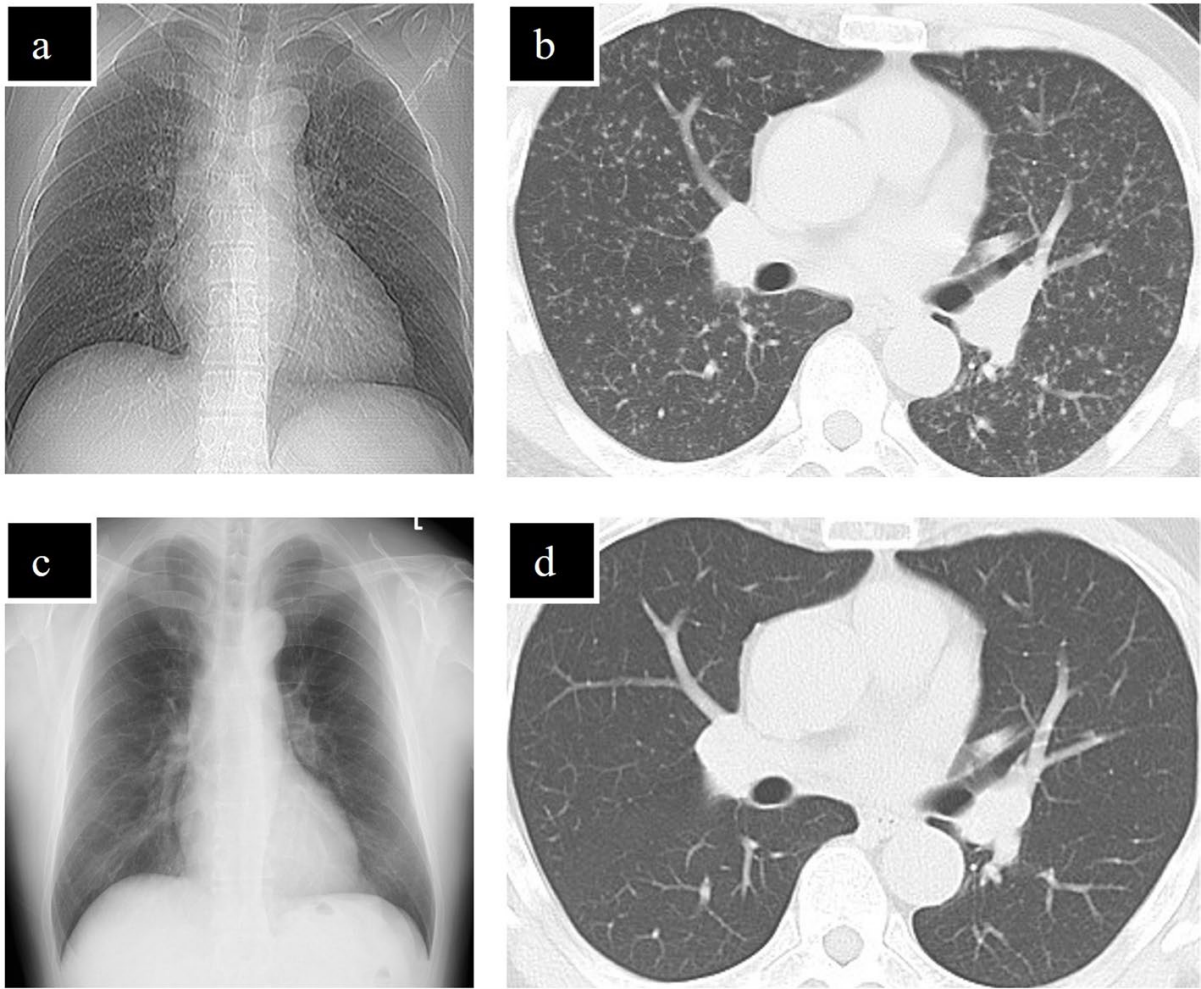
Chest X-ray and CT before and after treatment for miliary TB. Chest X-ray (a) and CT (b) images before treatment reveal multiple diffuse granular shadows in bilateral lungs and a lesion near the pleura with suspected hematogenous distribution. Chest X-ray (c) and CT (d) images after treatment show improvement of multiple diffuse granular shadows in bilateral lungs
